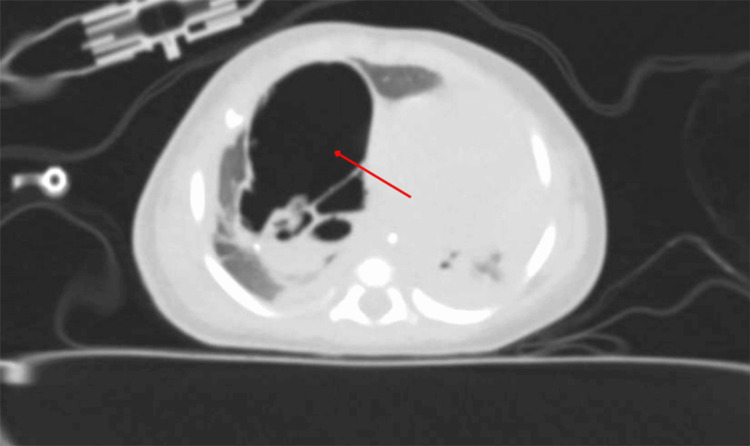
Contrast-enhanced axial chest CT at four weeks of age The red arrow points to a large, air-filled multicystic lesion occupying the right middle and lower lobes, causing displacement of the mediastinum to the contralateral side.
